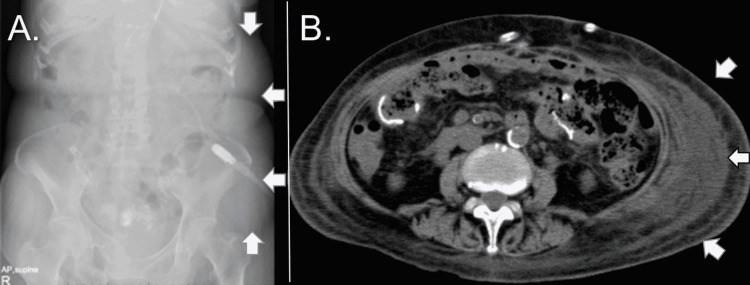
X-ray and CT images of subcutaneous edema after postoperative peritoneal dialysis resumption (case 3). (A) An abdominal X-ray shows the obviously low radiolucency area on the left abdomen (arrows). (B) The CT image shows subcutaneous edema spreading widely on the left lateral abdomen.
